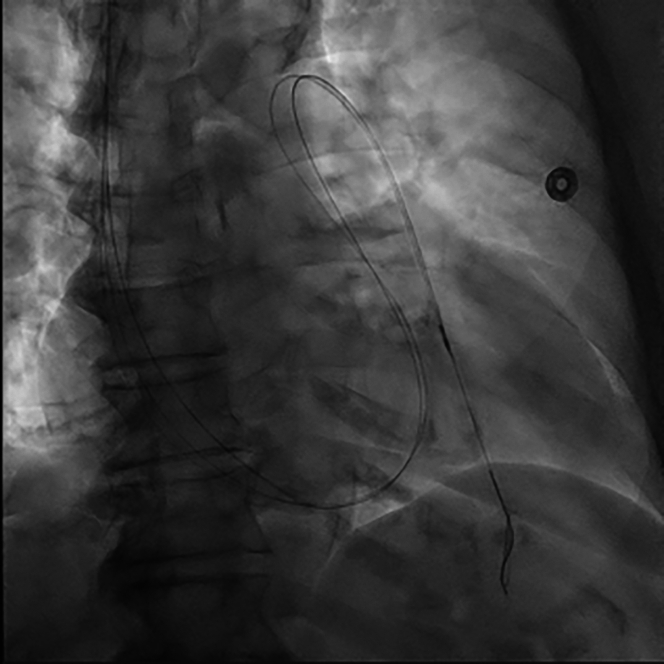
IVUS Catheter Advancing Into Distal LPA Target, With a Second Adjacent “Buddy Wire” Conferring Additional Support IVUS = intravascular ultrasound; LPA = left pulmonary artery.
